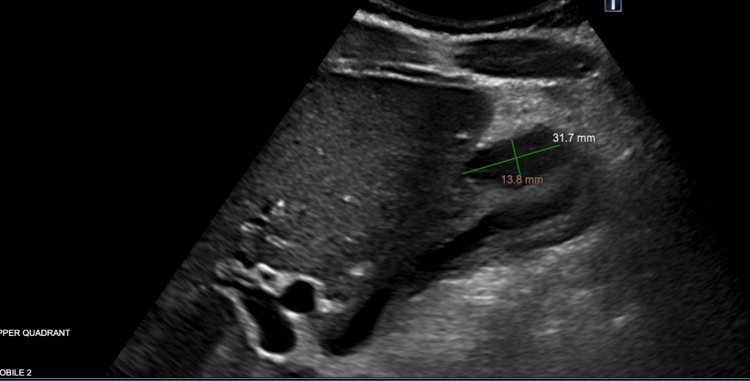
RUQ ultrasound two days later demonstrating possible gallbladder wall hematoma (13.6 mm × 31.7 mm) and wall thickening RUQ: right upper quadrant
